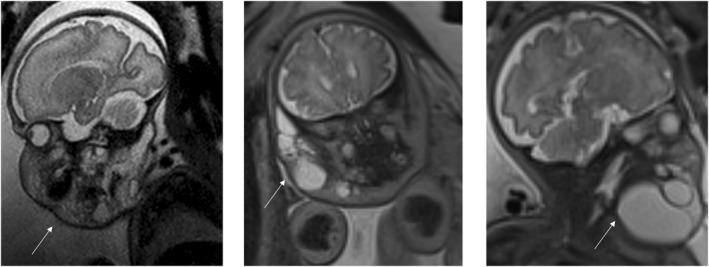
Fetal MRI images of lymphatic malformation. Left image ‐ T2 haste sagittal image of a 31 weeks fetus showing a multi‐septated cystic mass from orbit to upper thorax, which infiltrates into the face with airway compression. Middle image ‐ T2 haste coronal image of a 35 weeks fetus showing a septated multi‐cystic subcutaneous lesion right side of the face from orbit to neck. Right image ‐ T2 haste coronal image with head turned to the left of a 25 weeks fetus with an anterior cystic neck mass.
